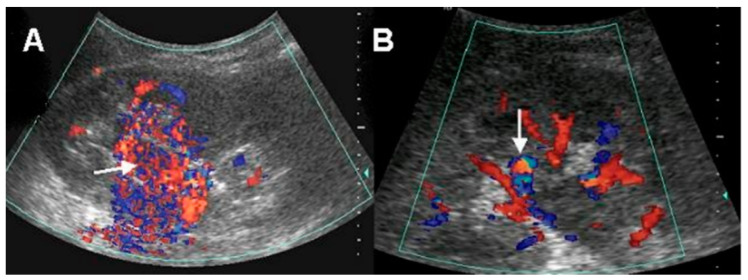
Doppler ultrasonographic findings: (A) conglomerated vascular structure (arrow) with blood-flow-rich area, (B) vascular turbulence (arrow).
